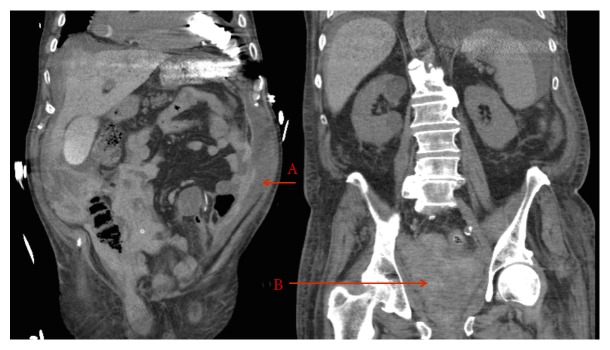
CT of abdomen and pelvis performed on POD11 demonstrating free fluid of mixed heterogeneity in the paracolic gutters (A) and pelvis (B) consistent with organizing hematoma.
